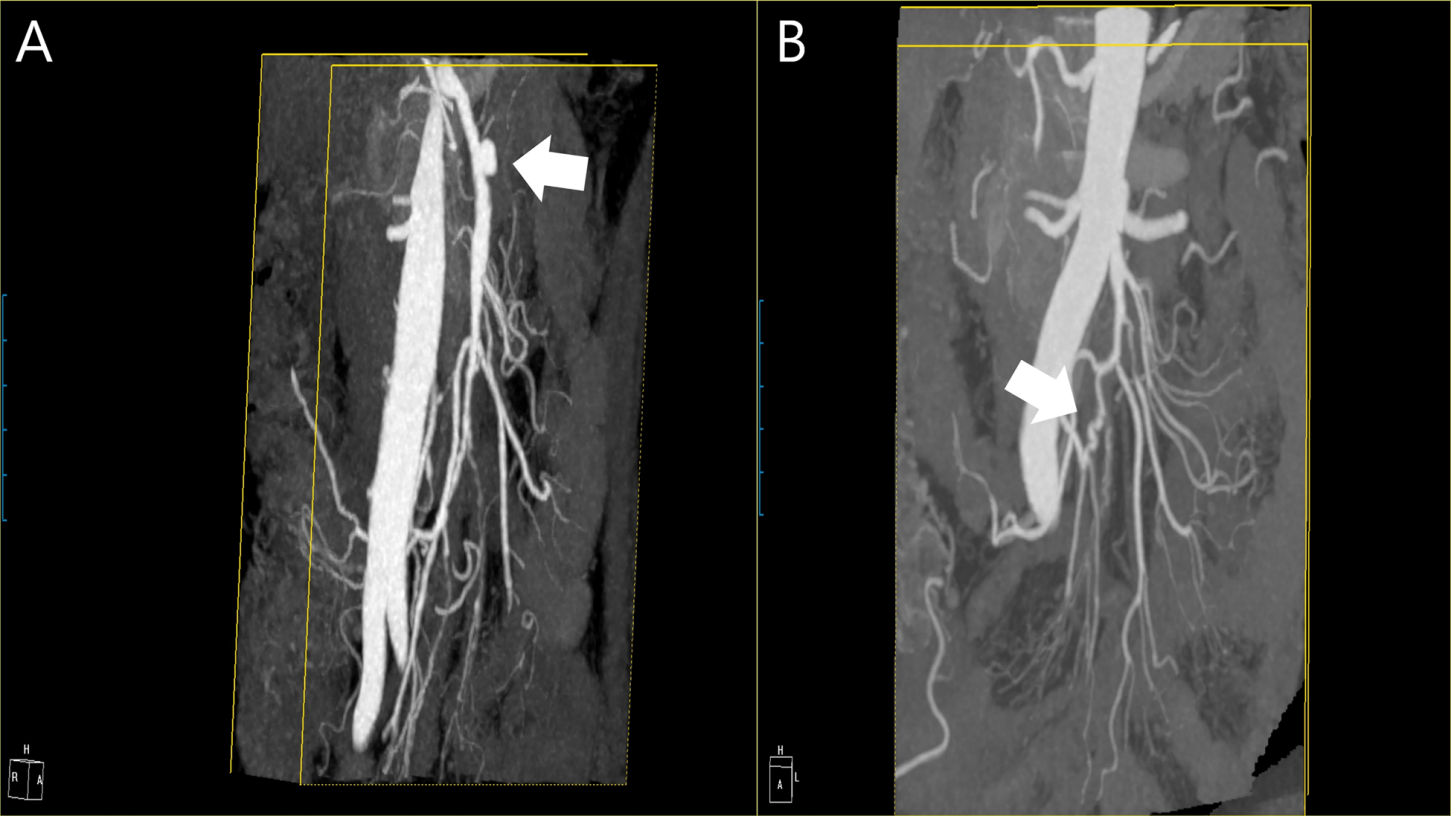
CT angiography (patient No. 6): (A) SMA dissection at presentation (in addition to right colic arterial dissection and occlusion of middle colic artery); (B) newly developed aneurysm of ileocolic artery seen 1 month after initial visit.
